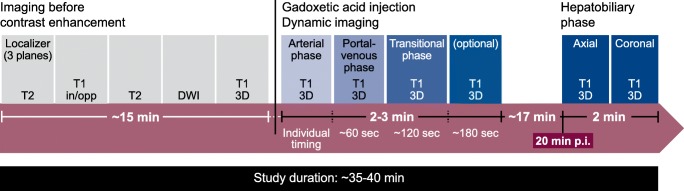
Traditional MRI protocol using gadoxetic acid—T1W, T2W, and DWI are performed before gadoxetic acid administration. DWI, diffusion-weighted imaging
